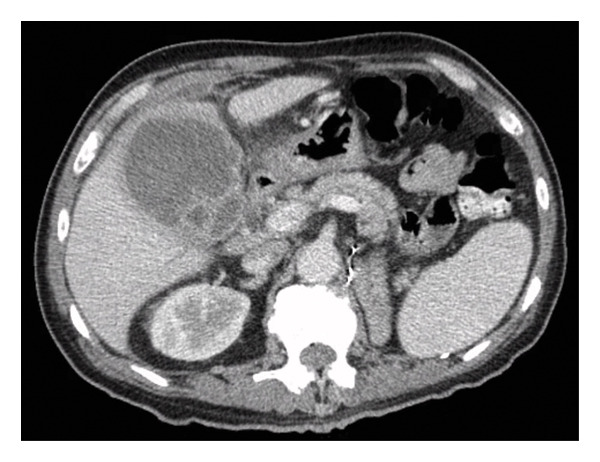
CT scan of the abdomen showing liver abscess.
